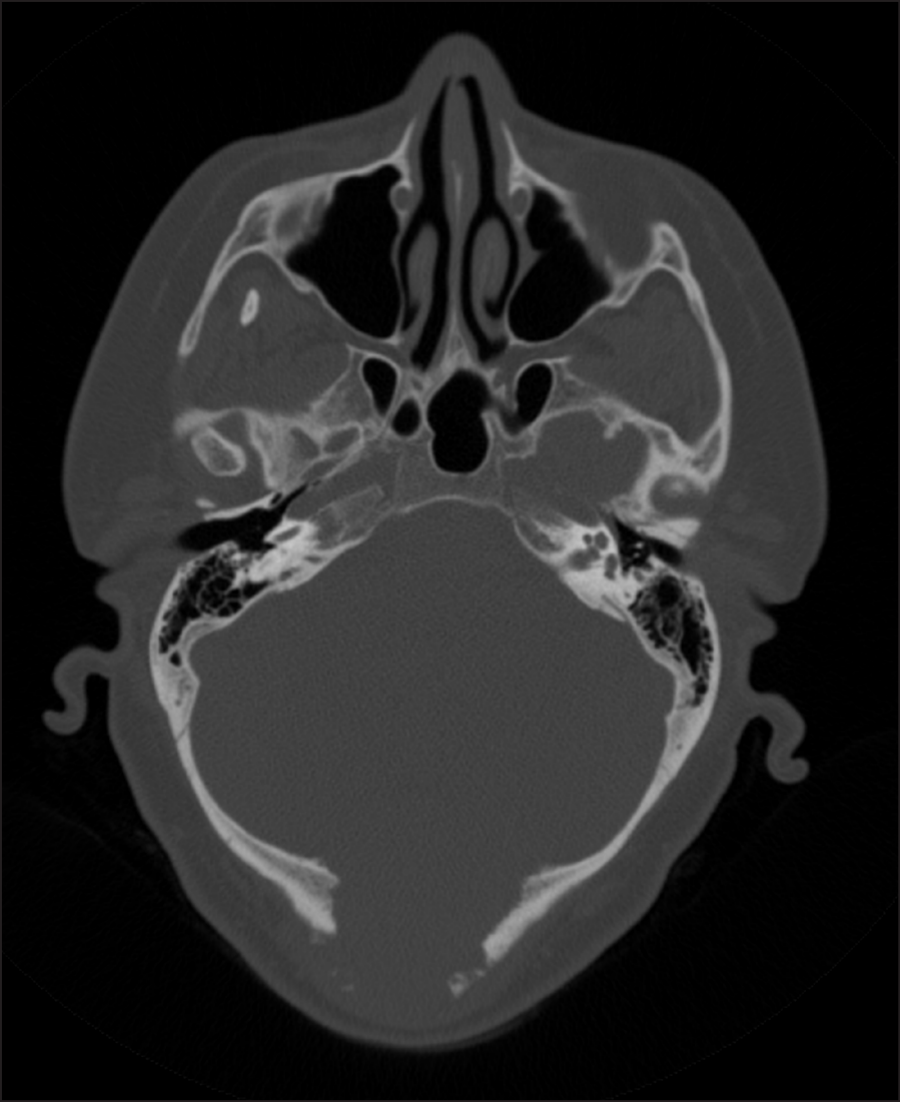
Axial CT reformat (bone window) depicts an extensive osteolysis centrally in the occipital bone with an outward bulging soft tissue mass.
